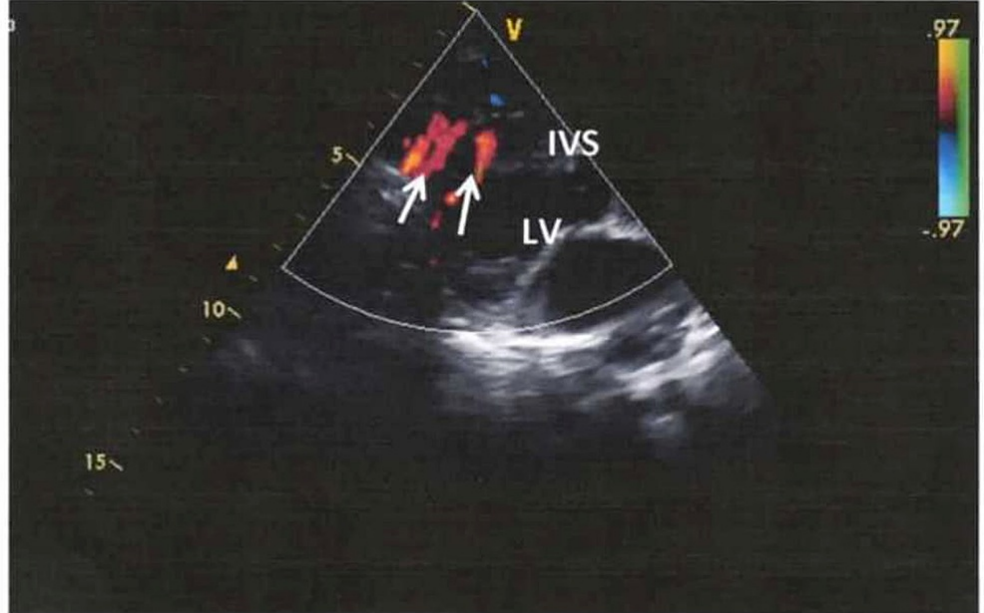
Echocardiogram (longitudinal axis). Colored flows (white arrows) are observed in the area of the interventricular septum (IVS), that correspond to collateral vessels.
